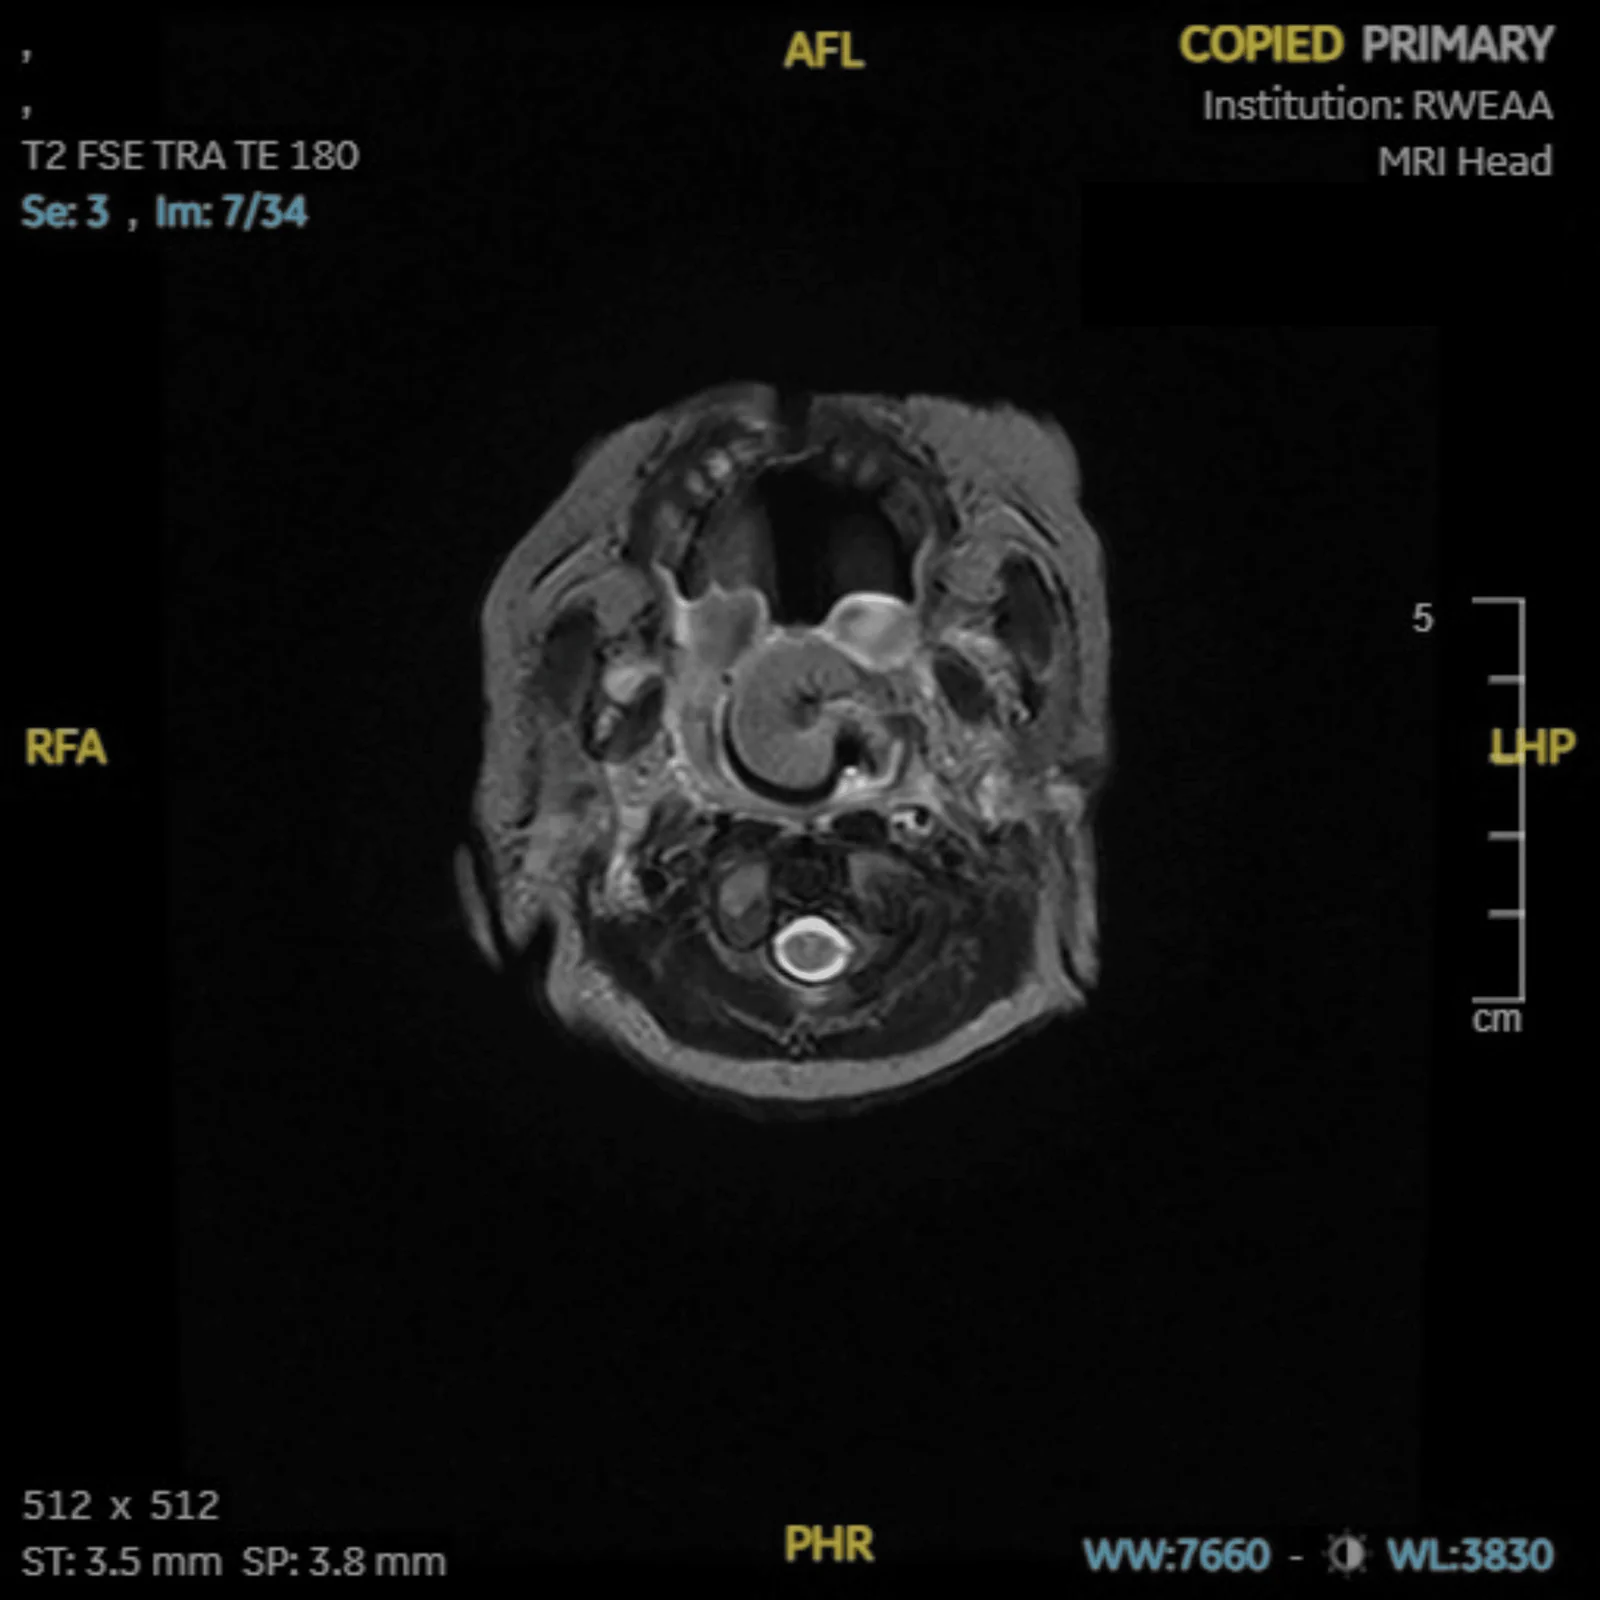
T2-weighted MRI scan showing hairy polyp (axial view) MRI: magnetic resonance imaging
